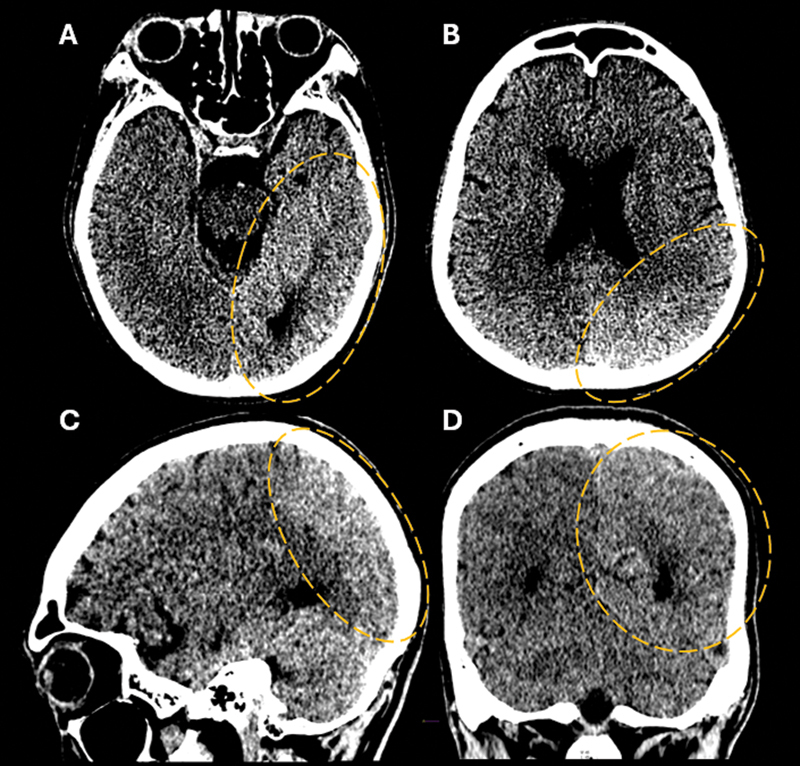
Noncontrast head computed tomography (CT). (
